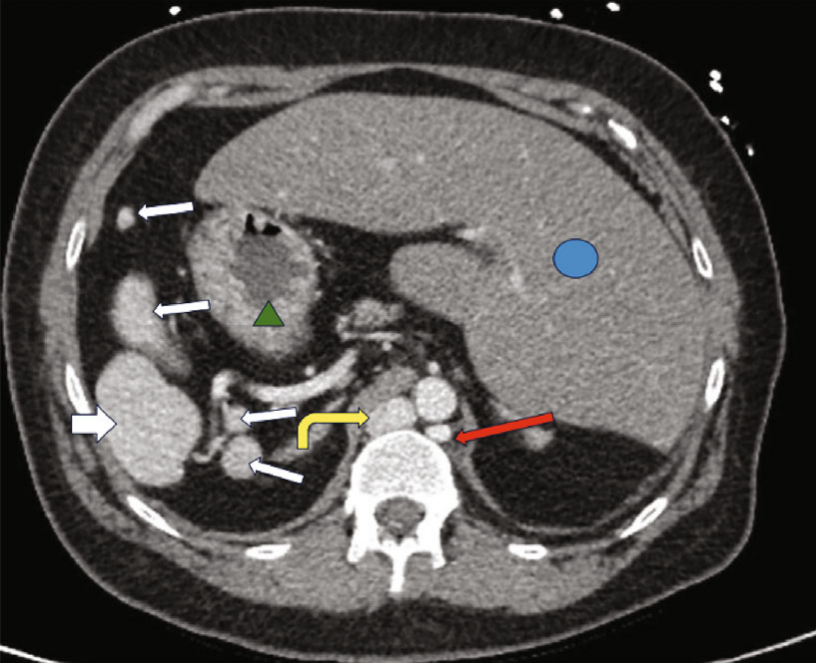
Axial noncontrast CT shows the liver in the LUQ (blue circle), the stomach in the RUQ (green triangle), polysplenia (white arrows), a circumaortic left renal vein (red arrow), and a prominent azygos vein (yellow curved arrow).
